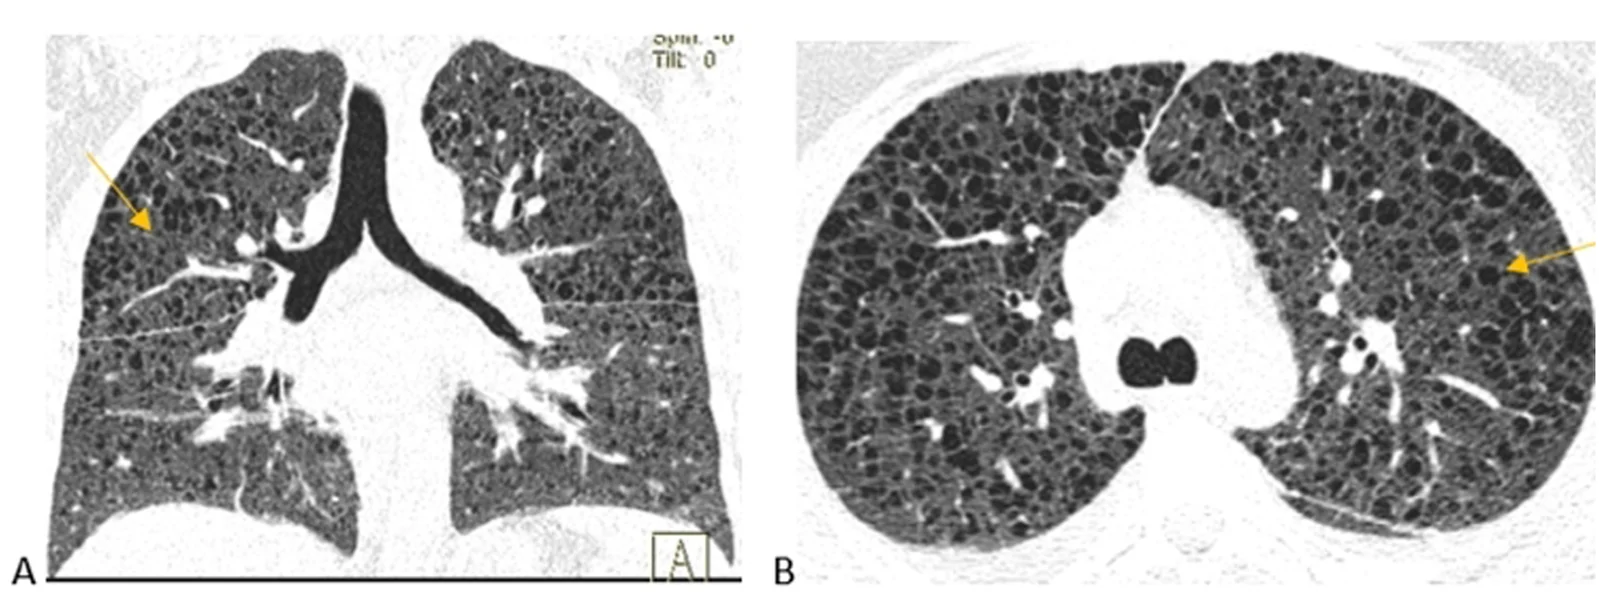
Chest CT lung window coronal (A) and axial (B) plane show bilateral symmetric numerous thin-walled cysts of similar size (arrows) with preserved normal intervening parenchyma.
